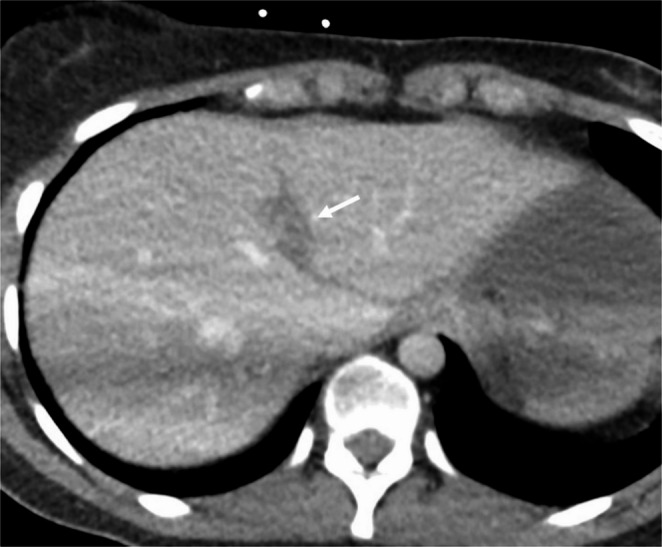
24-year-old female status-post motor vehicle collision. Axial post contrast CT demonstrates a laceration through the central liver (arrow) which was not described in the original outside report. The patient was managed conservatively
